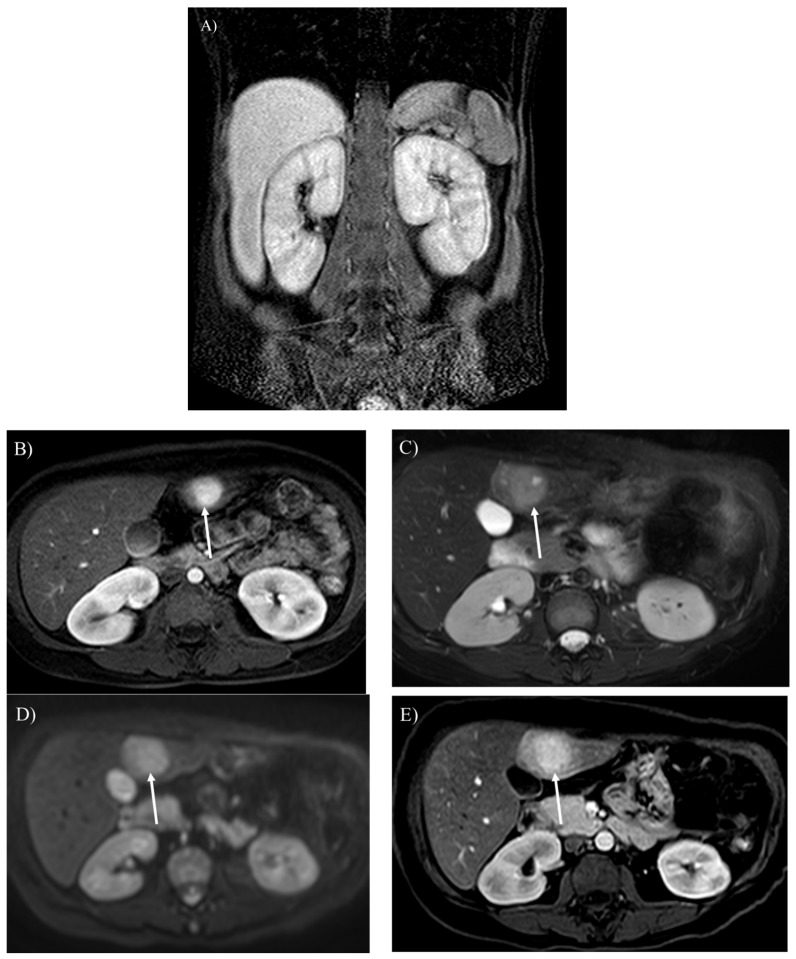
A 20-year-old woman with glycogen storage disease type Ia exhibiting enlargement of the kidney (A). Dynamic MRI (B) reveals a hepatic adenoma presenting strong enhancement in the arterial phase (arrow). The hepatic adenoma shows high intensity on T2-weighted (C) and diffusion-weighted imaging (D), (arrows). The tumor gradually enlarged over 13 years (E), (arrow).
